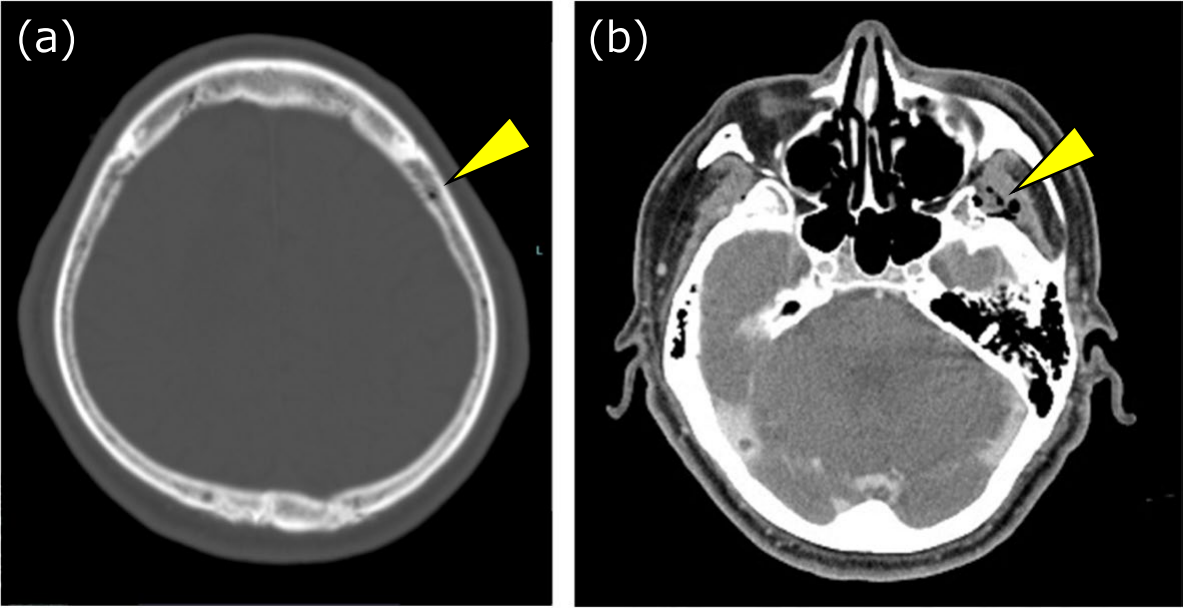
Postoperative CT findings in case 1. a CT brain image showing air densities in the diploic layer of the skull. b CT brain image showing air densities in the bilateral cervical regions
